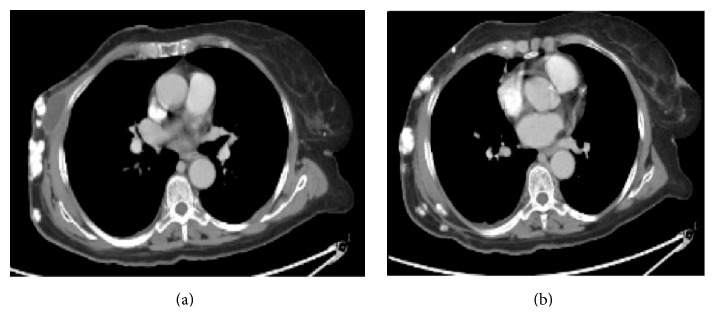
Axial contrast-enhanced postoperative CT performed after three months demonstrates postoperative changes including seroma and multiple right chest wall subcutaneous calcified nodules compatible with metastasis.
